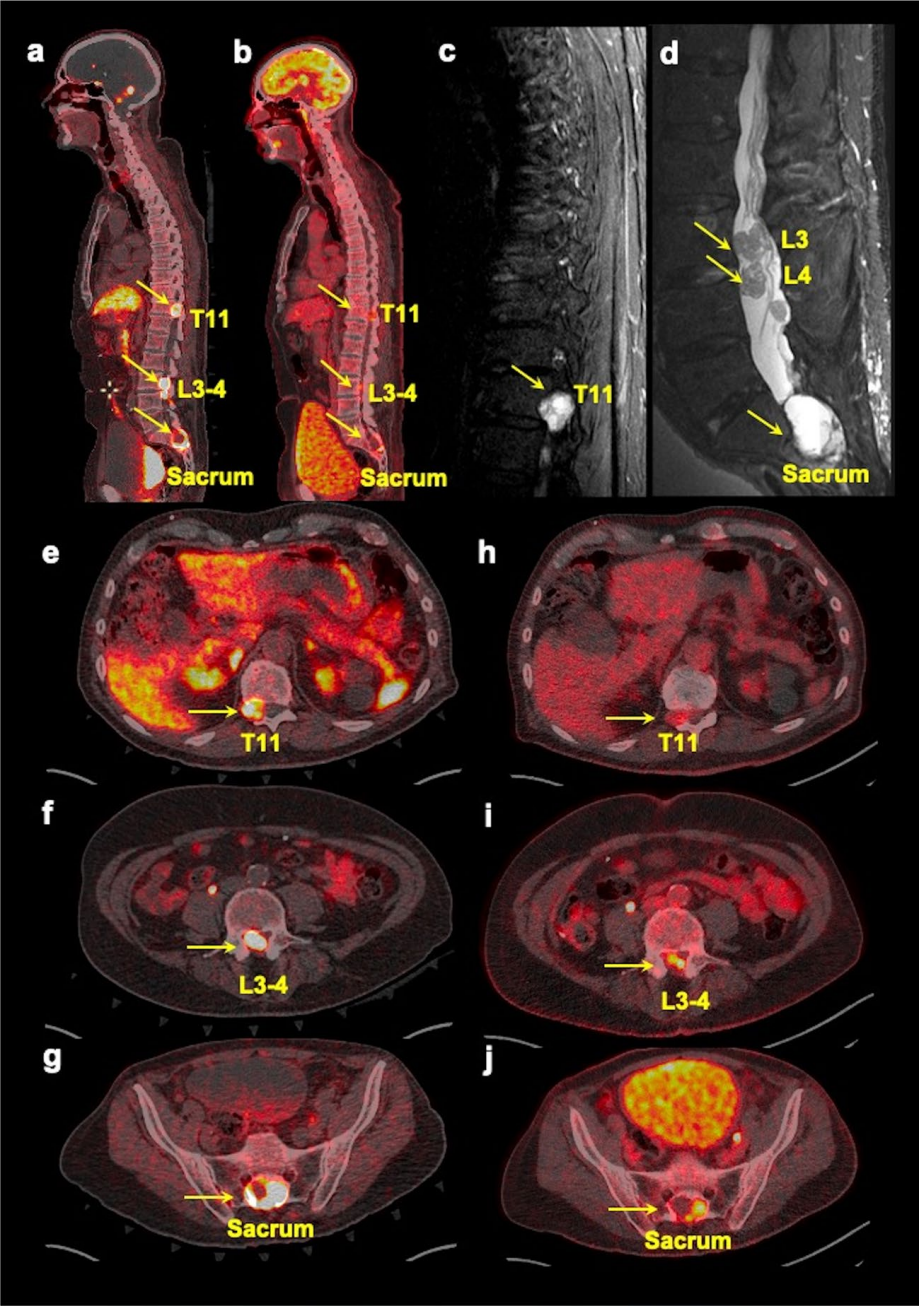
Multimodality imaging in a spinal bone metastatic pheochromocytoma/paraganglioma participant with spinal canal involvement. The whole-body fused sagittal (a, b) images of ^68^Ga-DOTA(0)-Tyr(3)-octreotate ([^68^Ga]DOTATATE, a) PET/CT, ^18^F-fluoro-2-deoxy-d-glucose ([^18^F]FDG, b) PET/CT, and sagittal short tau inversion recovery (STIR, c, d) images of thoracic (c), lumbar (d), and axial (e–j) fused PET/CT images of [^68^Ga]DOTATATE (e–g) and [^18^F]FDG (h–j) of a 71-year-old man with negative germline testing in pheochromocytoma and paraganglioma susceptibility genes. This figure shows spinal bone metastases (arrows, a–j) in T11, L3–L4, and sacrum with spinal canal involvement. In such cases, a dedicated MRI of the spine (showing intradural tumors, here) to derive more anatomic information should be obtained
